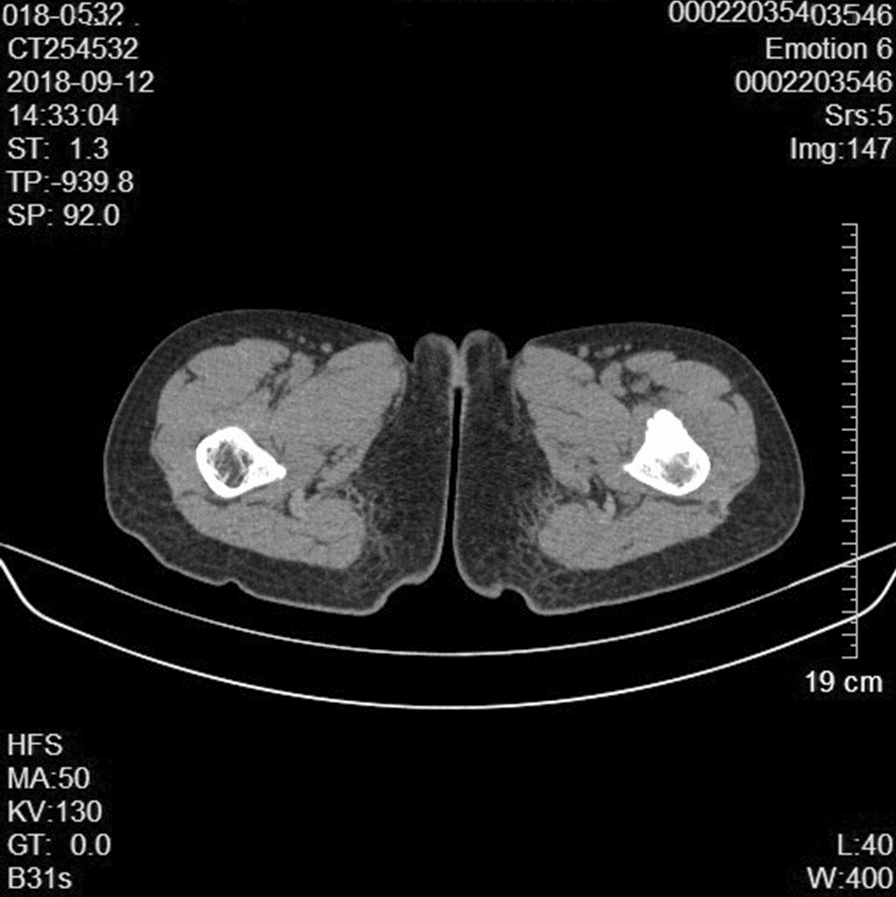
Preoperative CT displays thickened cortex in the anterior surface of the left proximal femur with specks of calcification and inhomogeneity of the adjacent bone marrow cavity
